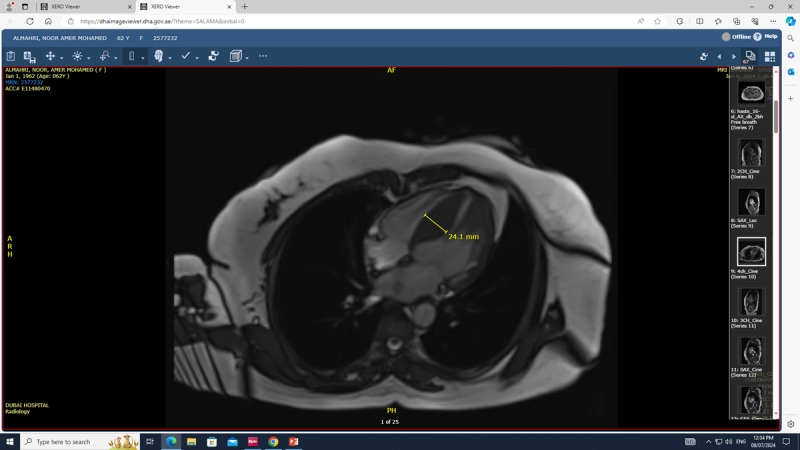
Figure 4. MRI Cranial 4 chamber view diastolic phase showing Hypertrophic Cardiomyopathy with Septum diameter measuring 24.1mm (yellow indicator).
